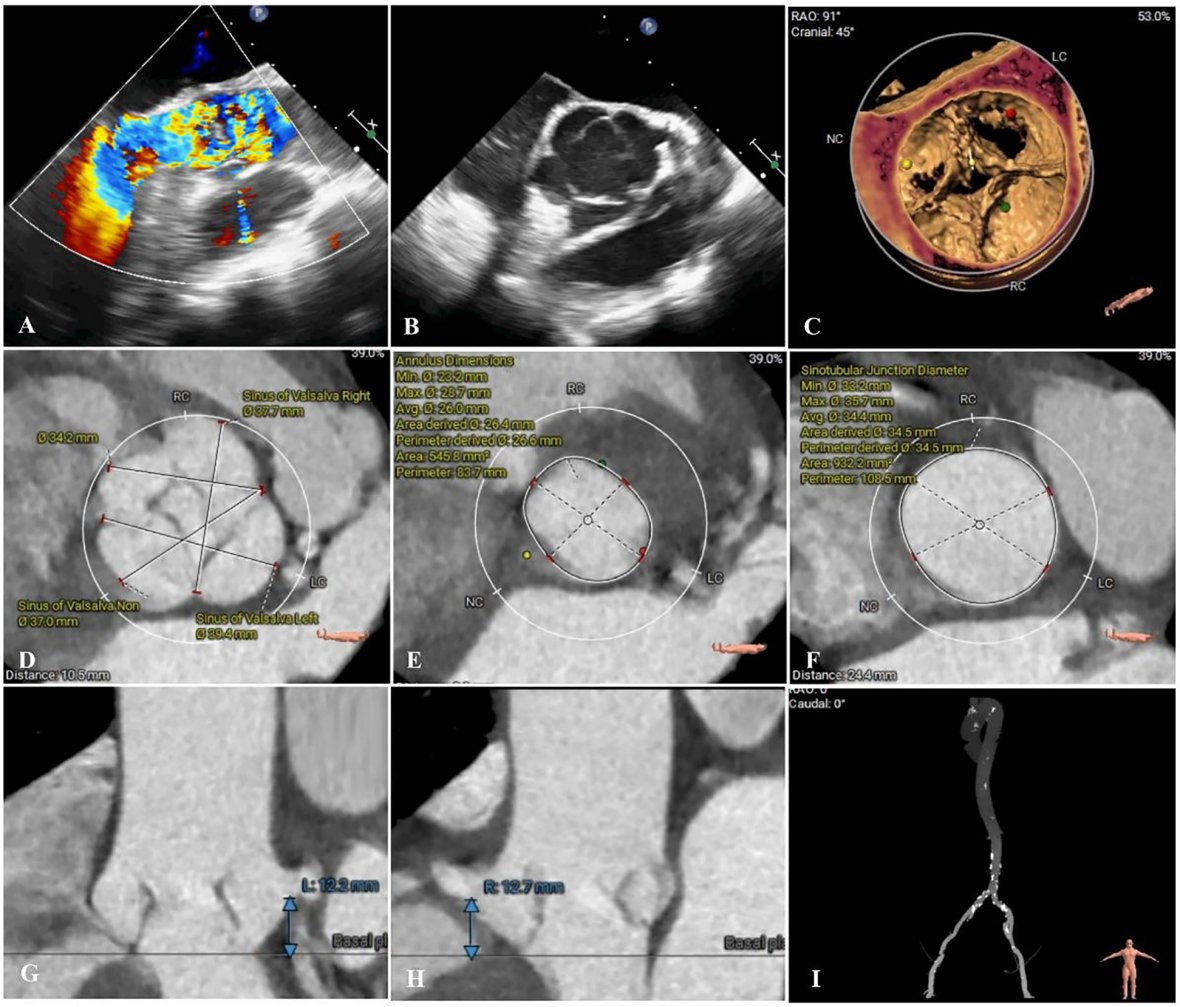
Preoperative imaging assessments. (A,B) Preoperative echocardiography revealed quadricuspid aortic valve and severe regurgitation (regurgitant jet area measuring 9.8 cm^2^). (C–I) Preoperative computed tomography angiography accessment. (D) Sinus of Valsalva diameters 37.0–39.4 mm; (E) Annulus diameter: 26.0 mm; (F) Sinotubular junction diameter 34.4 mm; (G,H) Left and right coronary ostia heights 12.2 and 12.7 mm.
